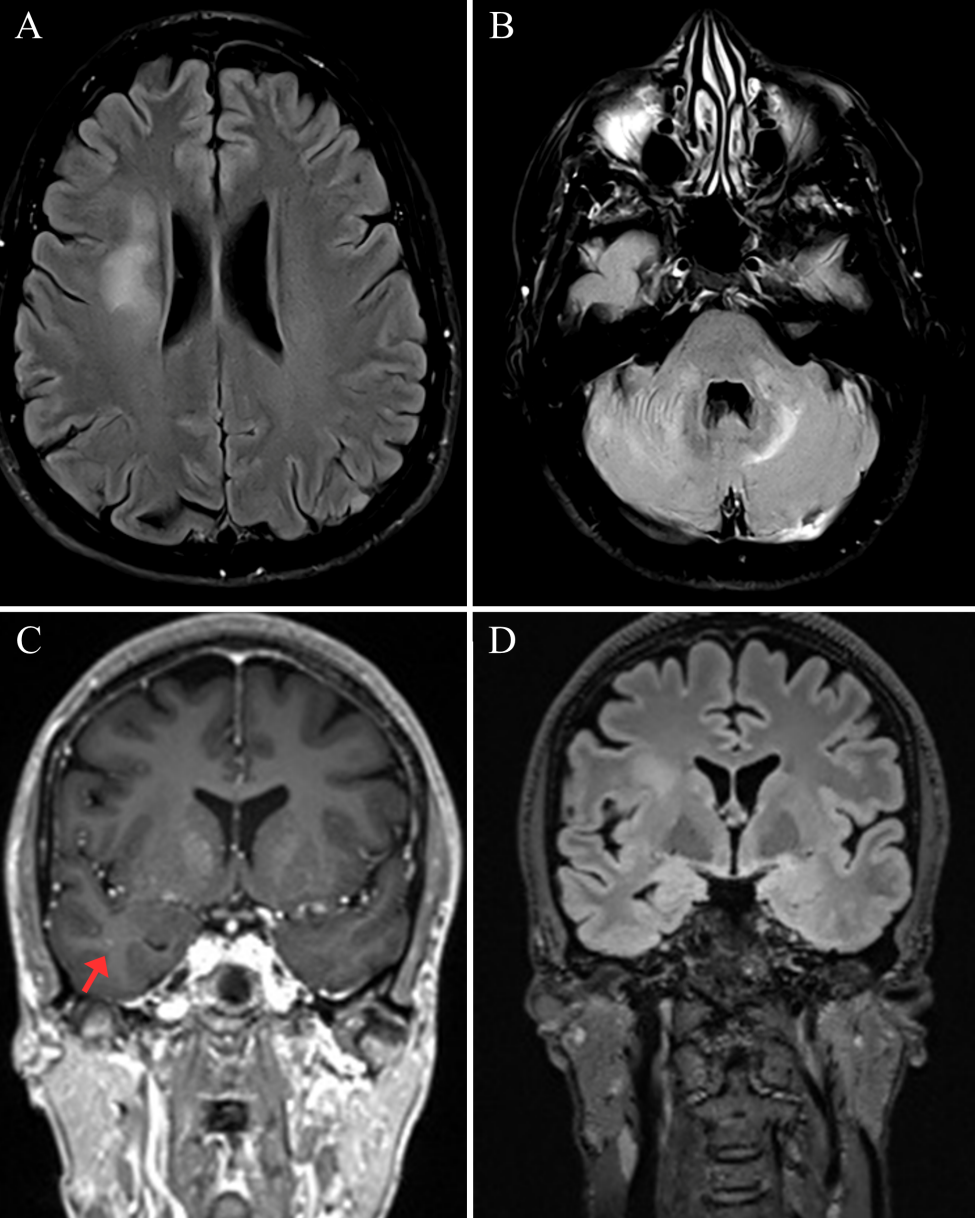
Widespread white matter lesions on brain magnetic resonance imaging (MRI). (A) Axial fluid-attenuated inversion recovery (FLAIR) MRI demonstrating an irregularly shaped hyperintense lesion in the periventricular and deep white matter of the right frontal lobe, suggestive of demyelinating or inflammatory etiology. (B) Axial FLAIR MRI showing hyperintensities in the cerebellum region, confirming an infratentorial component to the multifocal process. (C) Coronal post-contrast T1 at first MRI showing the lack of contrast enhancement in the large right frontal lesion (partially visible) and the presence of a small, avidly enhancing lesion in the right temporal lobe (red arrow), suggesting a focal area of active inflammation. (D) A coronal view demonstrating widespread T2/FLAIR hyperintensities involving the periventricular and deep white matter of the cerebral hemispheres, the temporal lobes bilaterally, and a moderate cortical atrophy, a pattern overlapping with encephalitis and various other central nervous system diseases, including manifestations of systemic autoimmunity.
